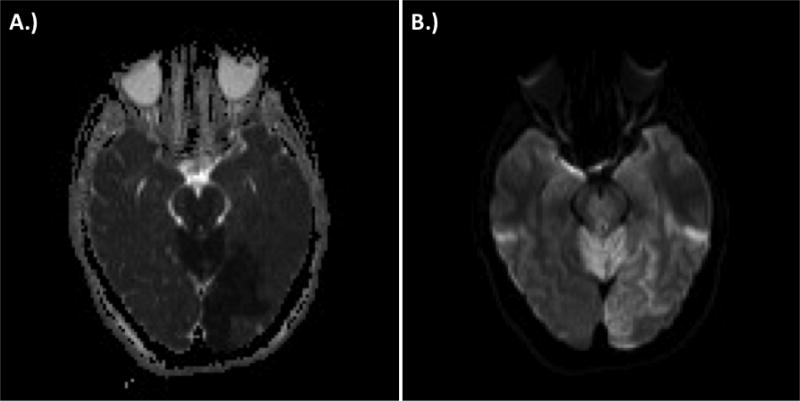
Immediate post-procedure magnetic resonance imaging demonstrating ischemic stroke within the cerebellum, brainstem, and left occipital cortex. A.) attenuated diffusion coefficient (ADC) sequence capturing left occipital stroke and B.) diffusion-weighted imaging capturing cerebellar, brainstem, and left occipital cortex stroke.
